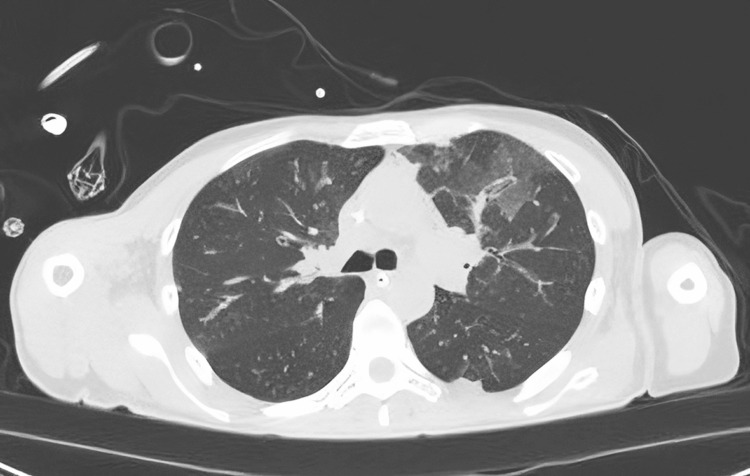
The chest CT showed the presence of multiple areas of ground glass hyperdensity and thickening of the pulmonary interstitium in the consolidative areas, a picture compatible with bilateral interstitial-alveolar pneumonia.
